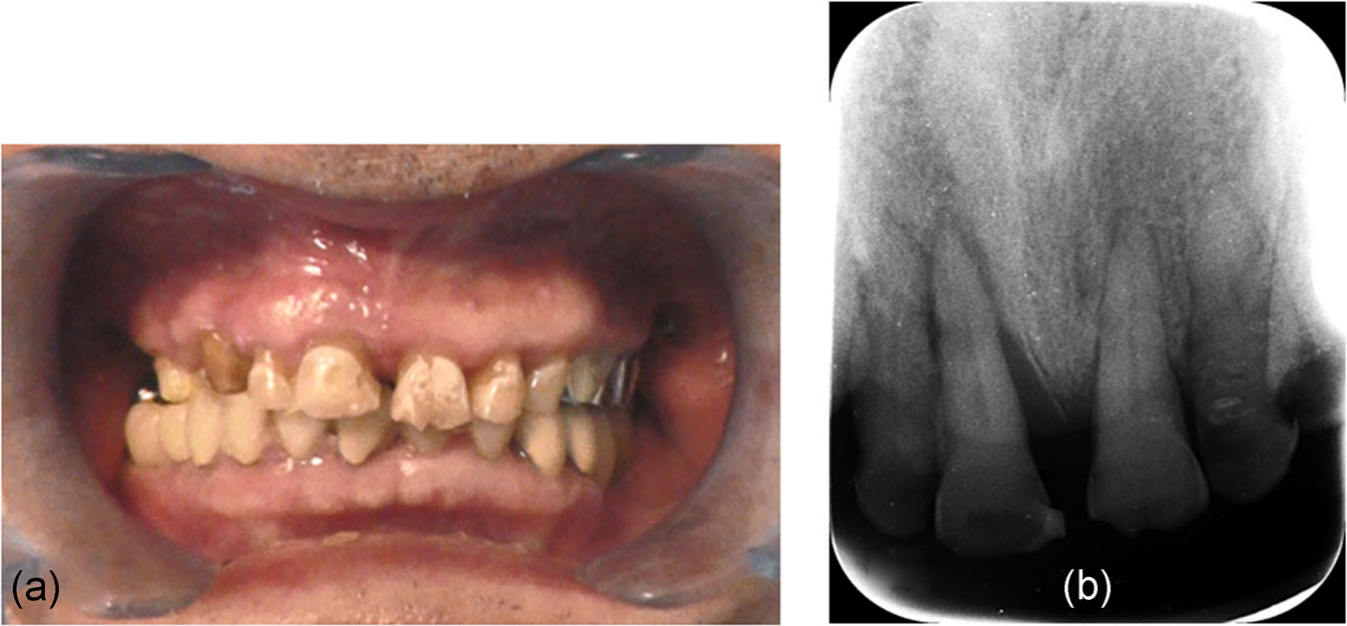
Oral fundings in Case 1; (a) intraoral anterior view, (b) intraoral radiograph
